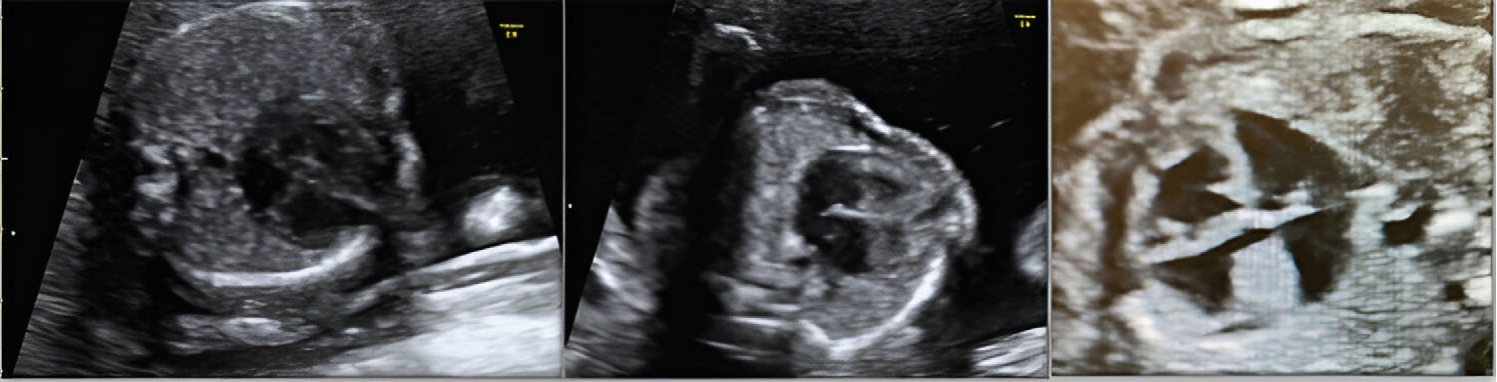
Echocardiogram performed during Week 22 of pregnancy.
